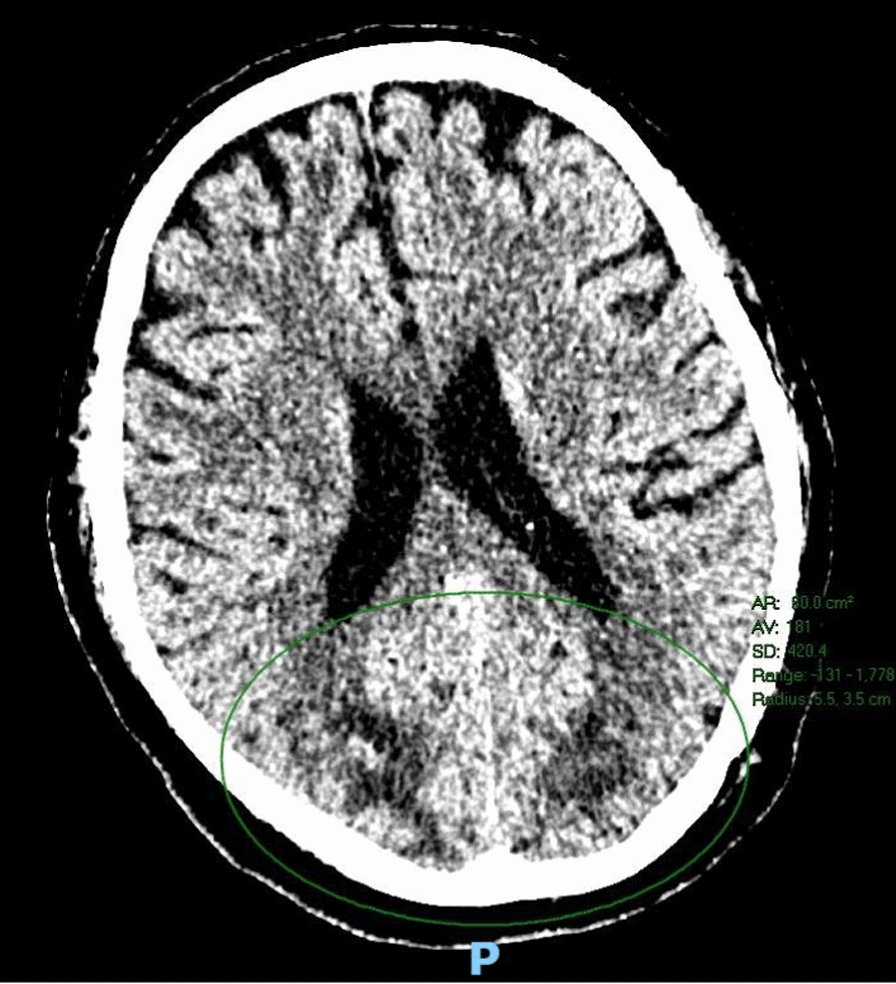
Computed tomography of brain perfusion (code-stroke) demonstrating abnormal predominantly subcortical hypoattenuation in bilateral occipital lobes (highlighted with a green circle) with new reduced blood mean transit time and increased cerebral blood volume. The radiological report did not quantify the mean transit time or cerebral blood volume
